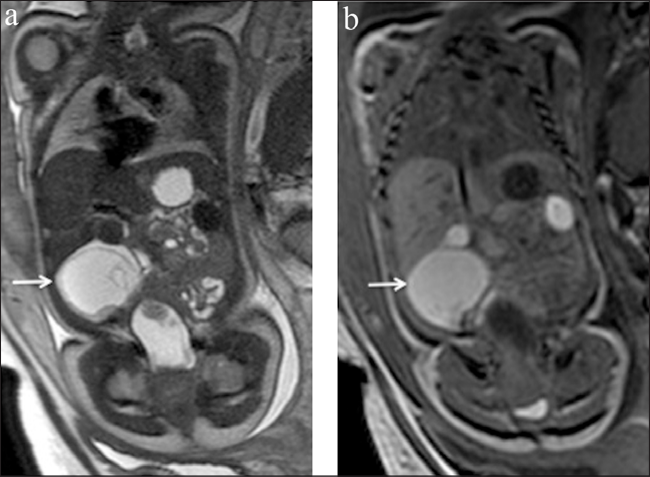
Characterisation of a pelvic cystic lesion-hemorrhagic ovarian cyst. (a) Coronal T2-weighted sequence on the abdomen of a 38 week-old female fetus presenting a hyperintense cystic lesion (arrow). (b) On the T1-weighted sequence slice performed at the same level, the lesion appears hyperintense. This T1 signal is highly suggestive of blood content leading to the diagnosis of a hemorrhagic ovarian cyst.
